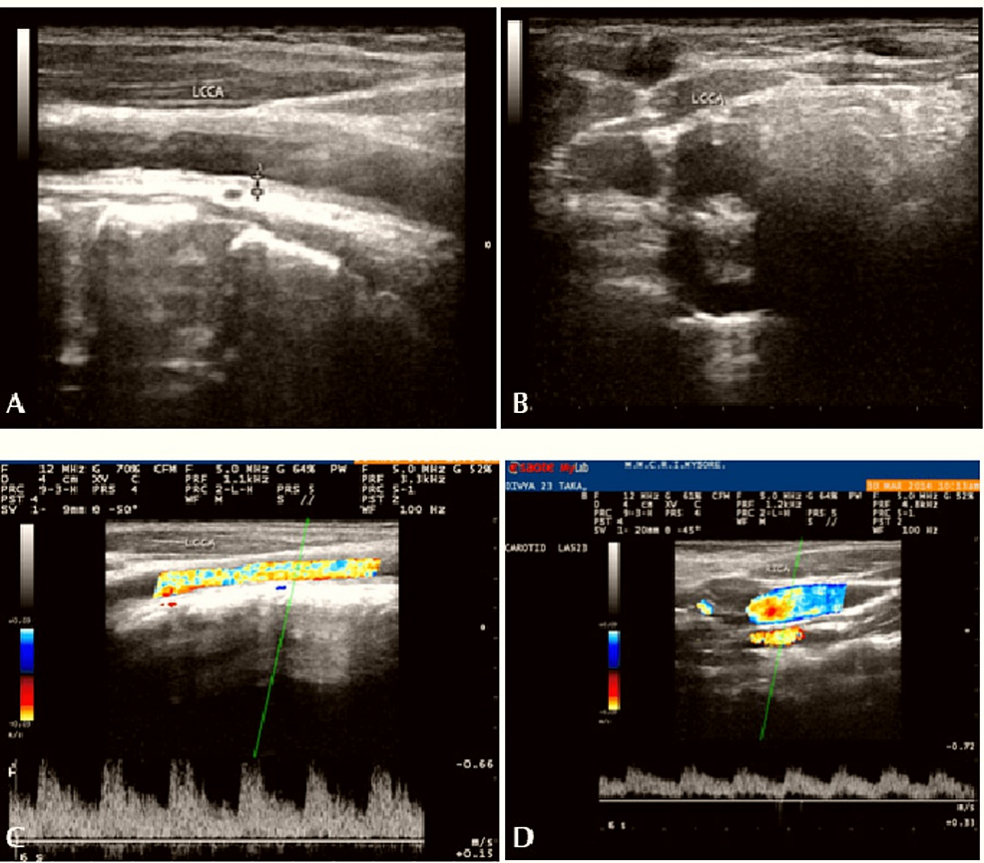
Left common carotid artery Doppler Carotid artery Doppler showing luminal narrowing in left carotid artery
